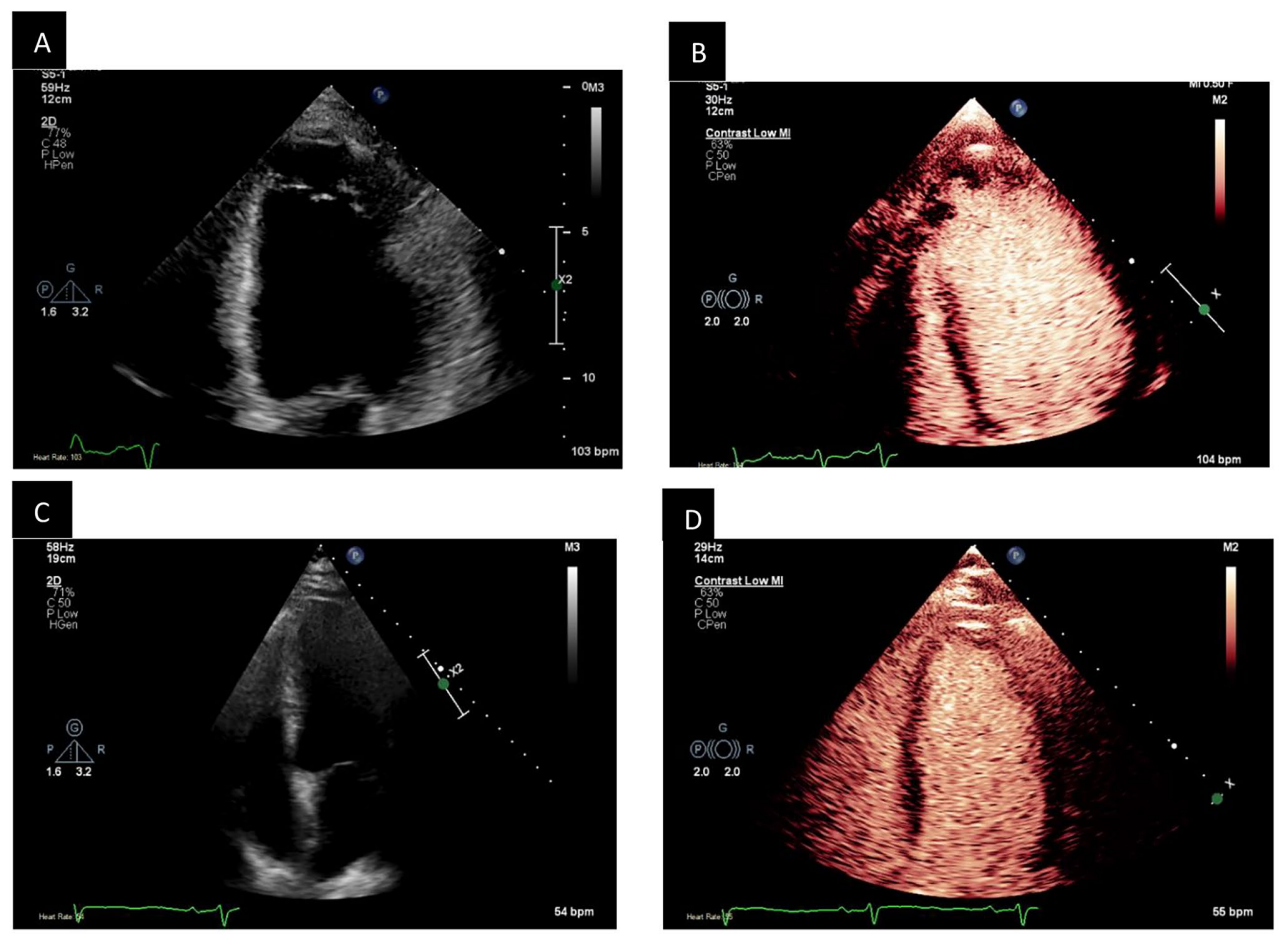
Examples of UEA utility (A) Apical 4 chamber view of a patient with akinetic apex with possible false tendons. Apical thrombus could not be excluded. (B) UEA demonstrates the presence of two apical thrombi. (C) Apical 4 chamber view of a patient with poor endocardial definition. Left ventricular ejection fraction could not be established. (D) UEA clearly defined the endocardial border leading to a diagnosis of globally reduced ejection fraction, 48% by Simpson’s biplane
